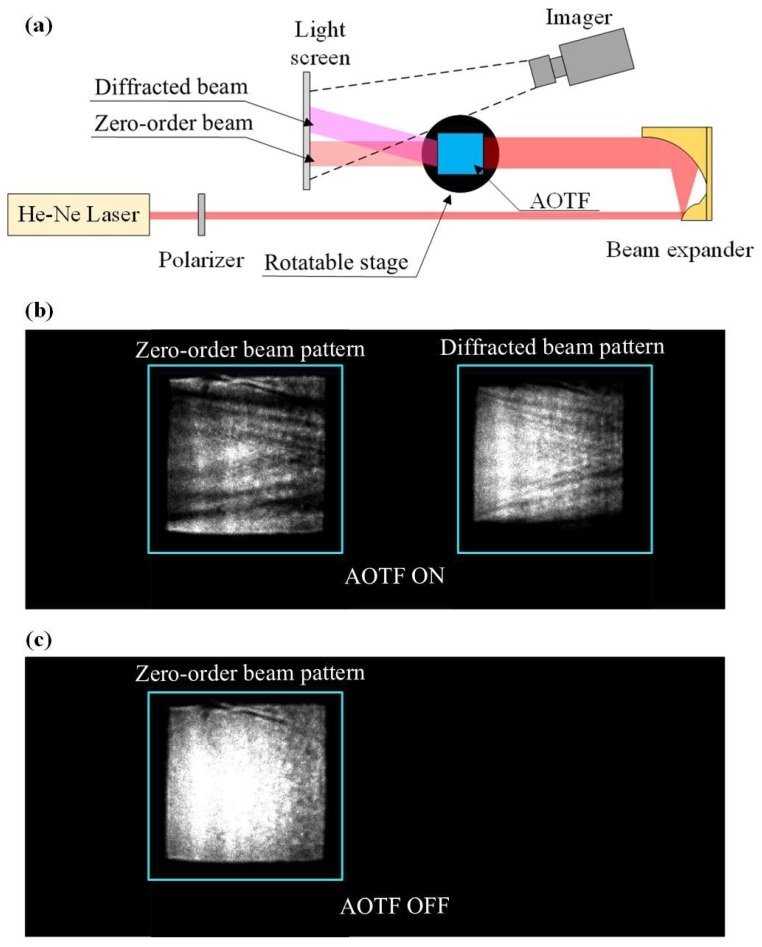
(a) Experimental setup. (b) Image of zero-order and diffracted patterns (under 50 MHz ultrasound frequency). (c) Image of zero-order pattern (AOTF off).
